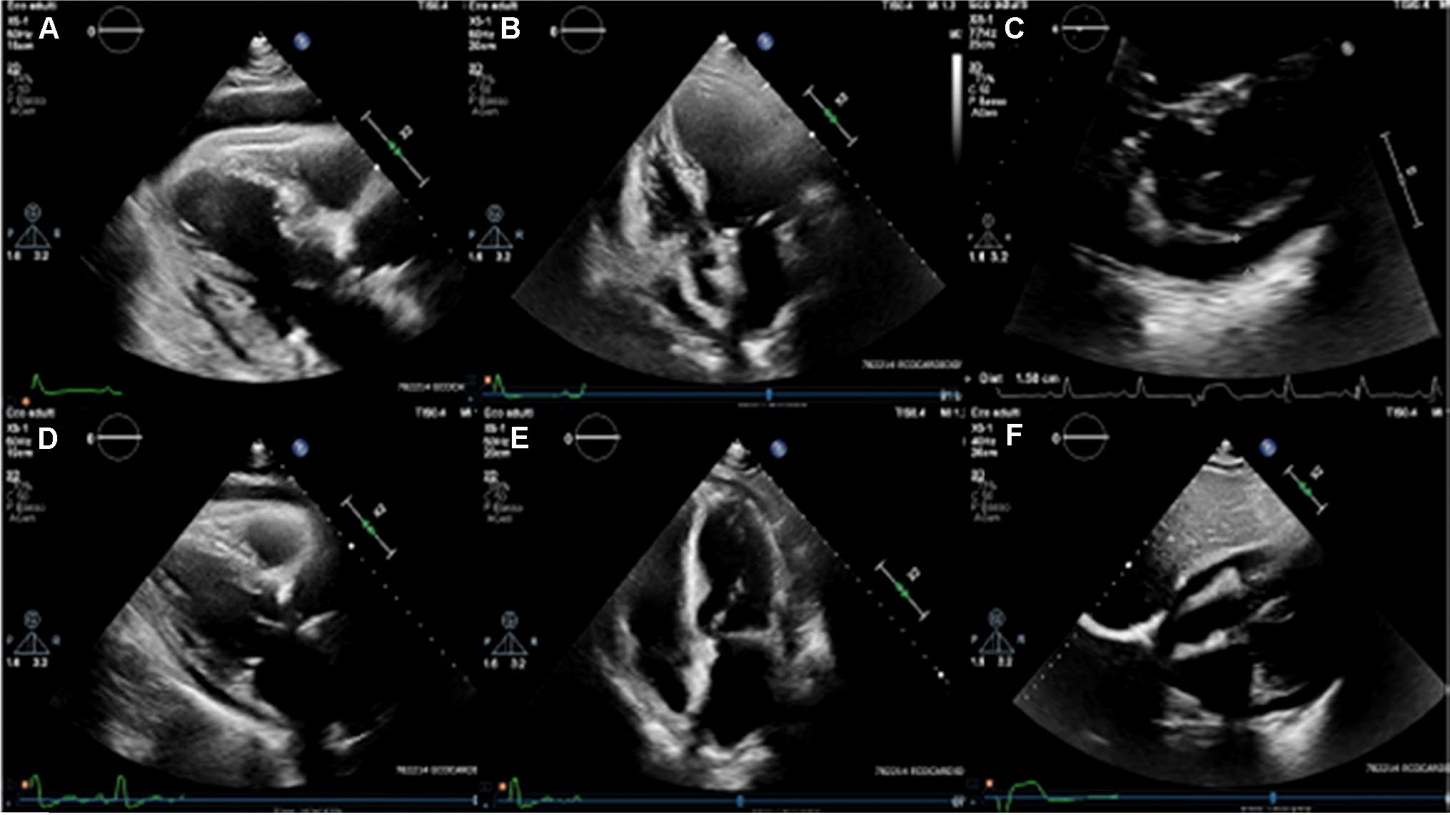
Echocardiographic assessment of pericardial effusion. a–c Diastolic frames of parasternal long axis, 4-chamber and subcostal views, respectively, acquired at our first evaluation. A moderately severe circumferential pericardial effusion is evident with signs of initial collapse of the right atrium. d–f The same views acquired after treatment with anakinra was started: pericardial effusion appears substantially reduced with no more signs of right atrium collapse
